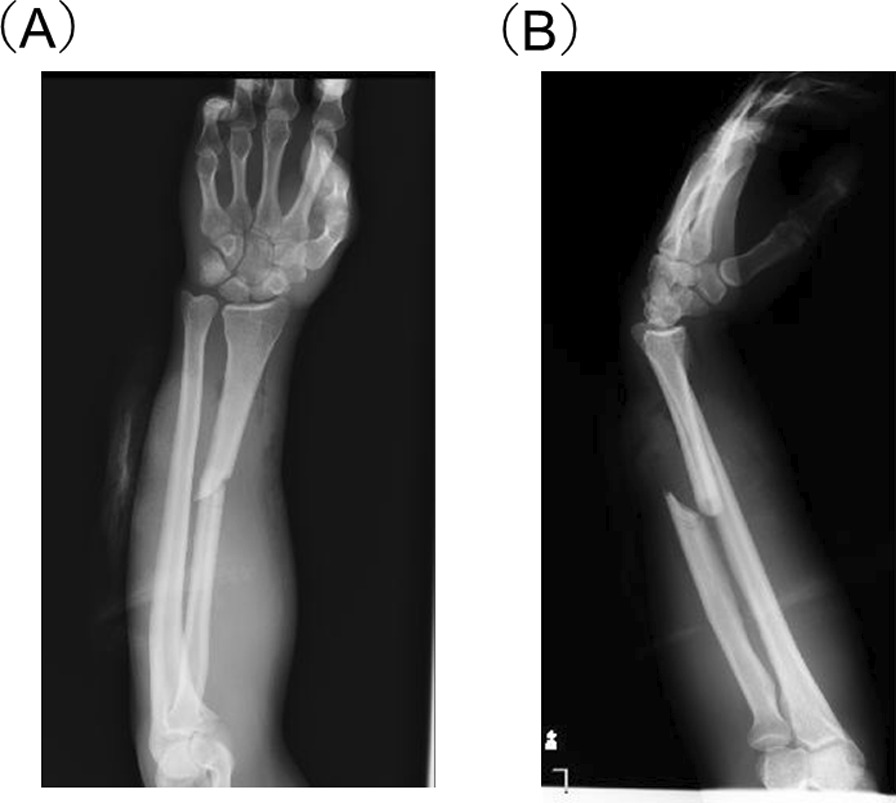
Conventional radiograph showing isolated radial shaft fracture. A Anteroposterior view, B lateral view
